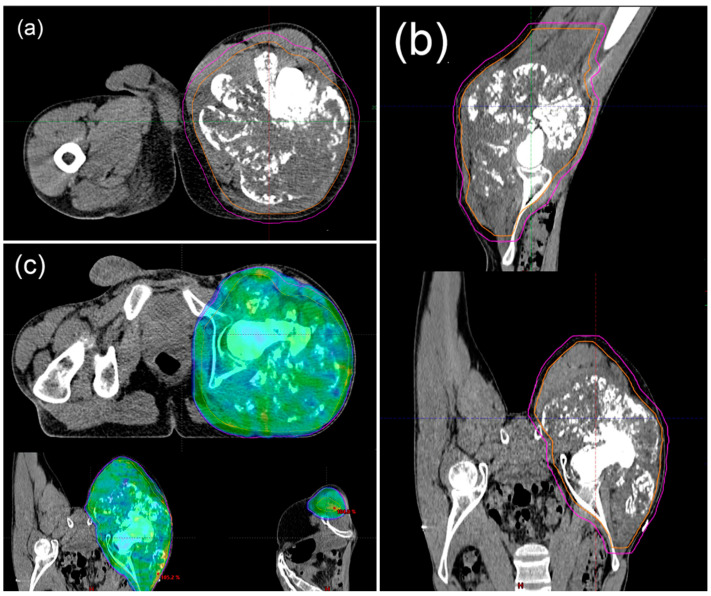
Nineteen-year-old patient diagnosed with dedifferentiated chondrosarcoma on the right thigh with lung metastases. Status after seven courses of chemotherapy with significant regression of lung lesions. Patient received radiotherapy for a lesion on the right thigh. Radiation therapy CT planning (a,b). Radiation therapy plan (c).
